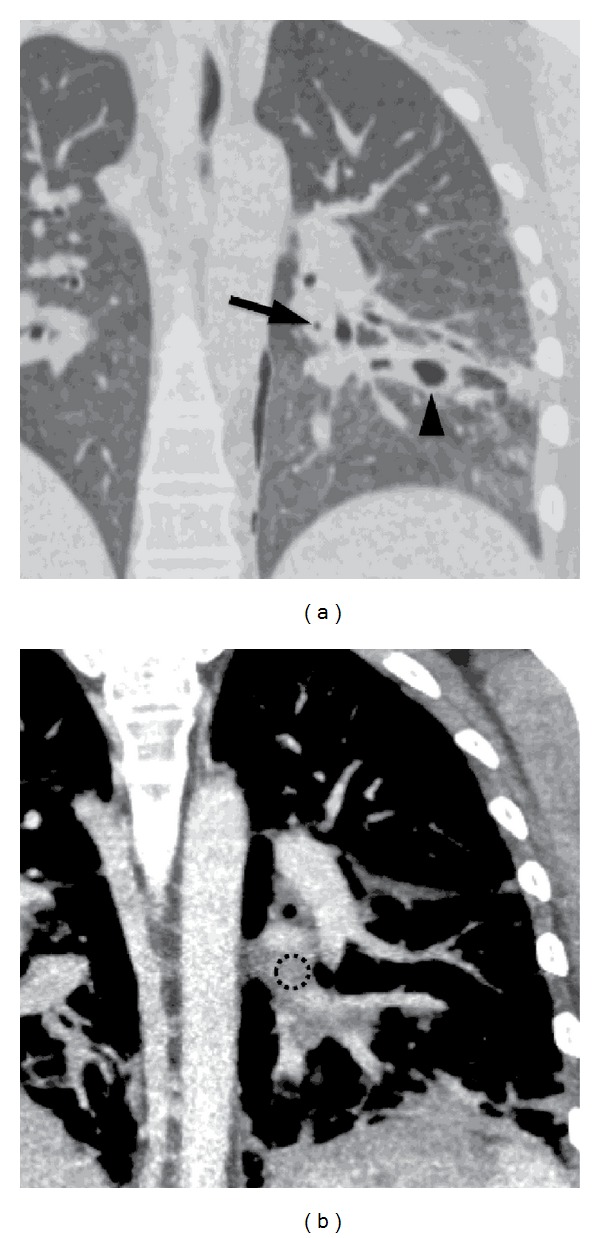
The lungs showing a focus of air in the pulmonary vein (arrow in (a)) and cavitary lesion (arrowhead). (b) is a CT with contrast performed earlier to show that the air is located in a pulmonary vein (dashed circle).
